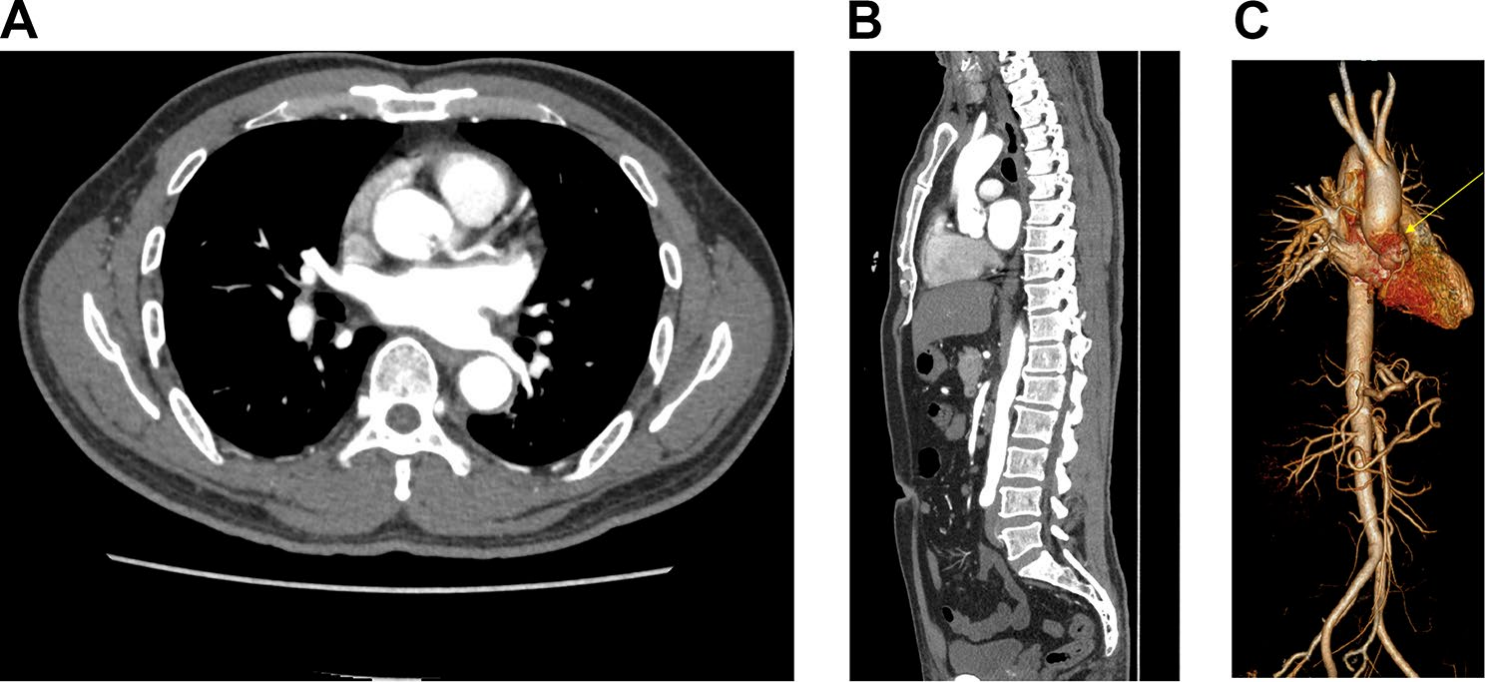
(A) and (B): The pursued CTA of aorta showed patency of coronary artery. The original thrombus decreased in size and became indistinct at the edges after the short-term anticoagulant and dual antiplatelet therapy. (C) Three-dimensional reconstruction of the aorta. Yellow arrow indicates the dissolving thrombus
